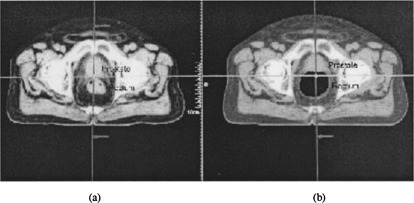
(Color) A prostate patient. (a) was from the planning CT, while (b) was from the treatment CT. The portal images did not indicate any movement, but the CT images showed clearly that the rectal content had changed significantly the posterior border of prostate relative to the isocenter.
